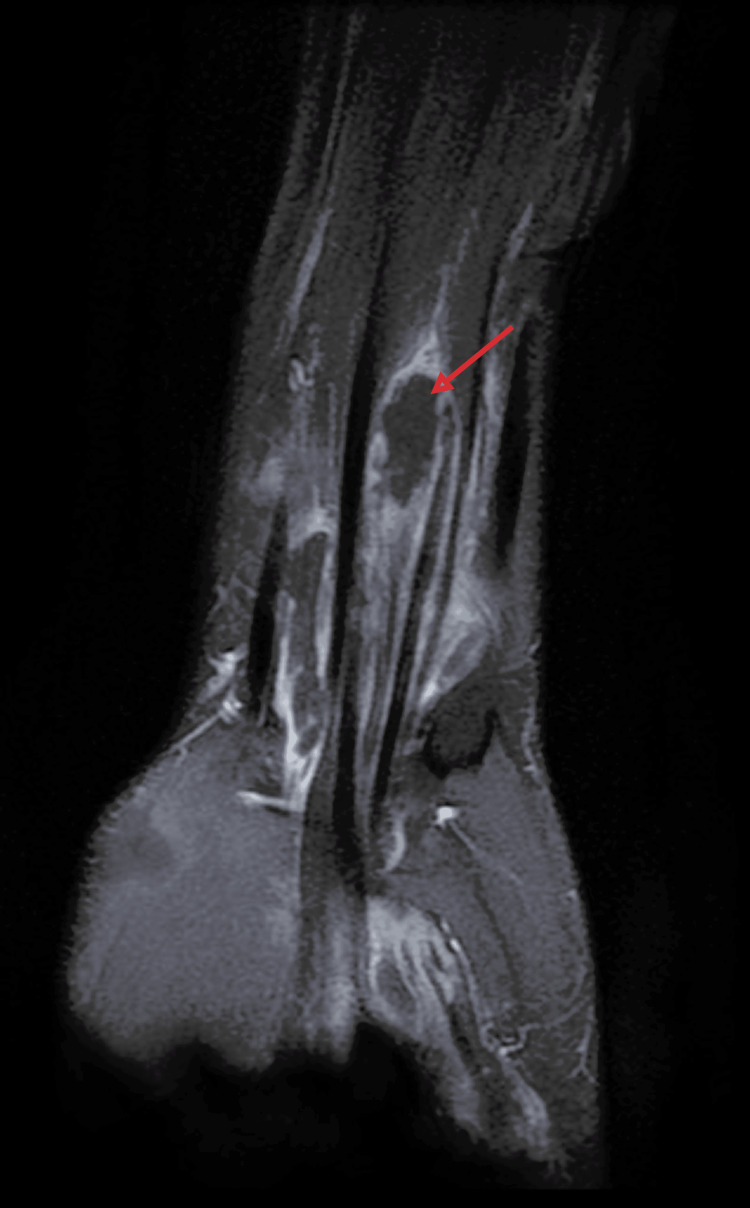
T1 fat-saturated coronal MRI of the wrist The image is showing fluid collection along the flexor tendon sheath, suggestive of tenosynovitis.
